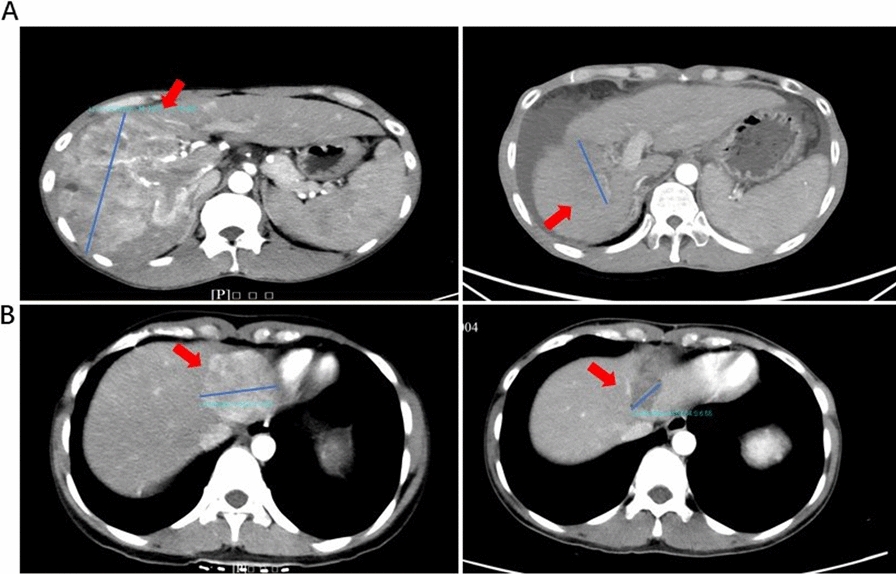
Representative images of liver tumor size in patients before and after lenvatinib treatment. A A CT image of a male patient before lenvatinib monotreatment (left) and 23 months after lenvatinib treatment (right). B A CT image of a female patient before treatment (left) and 4 months after lenvatinib + PD1 + HAIF treatment (right)
